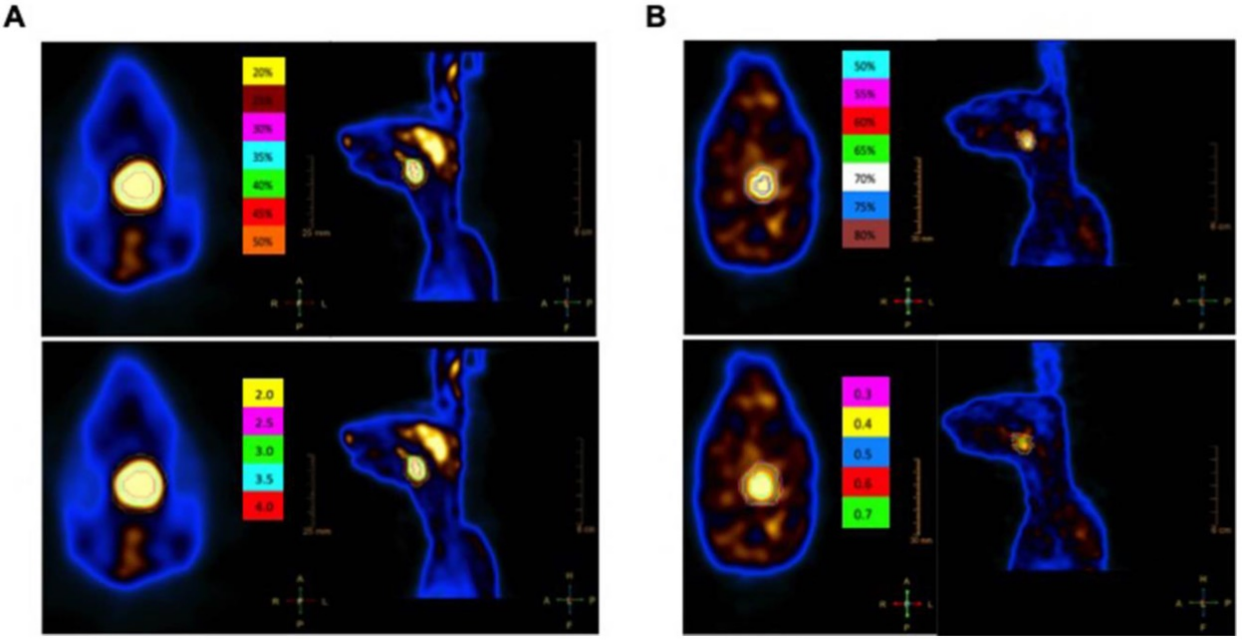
The GTV automatically segmented based on different SUV threshold values on ^18^F-FDG PET/CT (A) and ^18^F-FLT PET/CT (B). The ^18^F-FDG PET/CT images (Panel A) GTV_FDG2.0_, GTV_FDG2.5_, GTV_FDG3.0_, GTV_FDG3.5_, and GTV_FDG4.0_ were automatically segmented on the PET images using gradient-based methods, fixed threshold values at 2.0, 2.5, 3.0, 3.5, and 4.0 SUV, respectively. The axial and coronal images of GTVs segmented based on different SUV threshold values at 50%, 55%, 60%, 65%, 70%, 75%, and 80% of SUVmax. Also, the axial and coronal MRI images of GTVs segmented based on different SUV threshold values at 0.3, 0.4, 0.5, 0.6 and 0.7 SUV, receptively (panel B).
